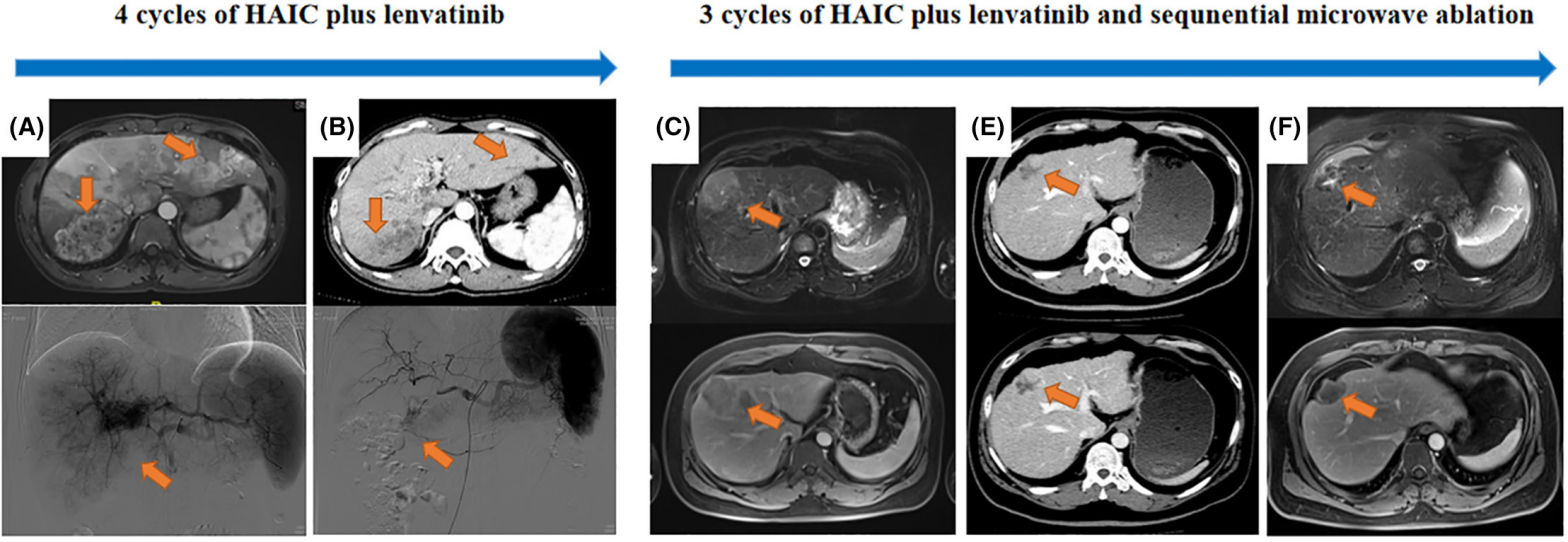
Examples of follow‐up medical records of H‐L and H‐L‐A treatment in Ad‐HCC. A 57‐year‐old female patient with HCC (triangle) were examined (The maximum diameter, 8.5 cm; AFP, 4258 ng/mL) by contrast MRI scanning on April 2019 (A). The tumor (triangle) has shrunk significantly after four‐cycle HAIC and lenvatinib treatment and 1 year later, the tumor is completely necrotic and AFP level is norma (B). A 32‐year‐old male patient with HCC (triangle) were examined (The maximum diameter, 5.6 cm; AFP, 845 ng/mL) by contrast MRI scanning on August 2018 (C). The tumor (triangle) has shrunk significantly after three‐cycle HAIC combined with lenvatinib (The maximum diameter, 1.8 cm; AFP, 8.6 ng/mL) (D) and the sequential MWA were used to eradicate completely the lesion and coagulation necrosis zone was shown (triangle) after half a year (E).
